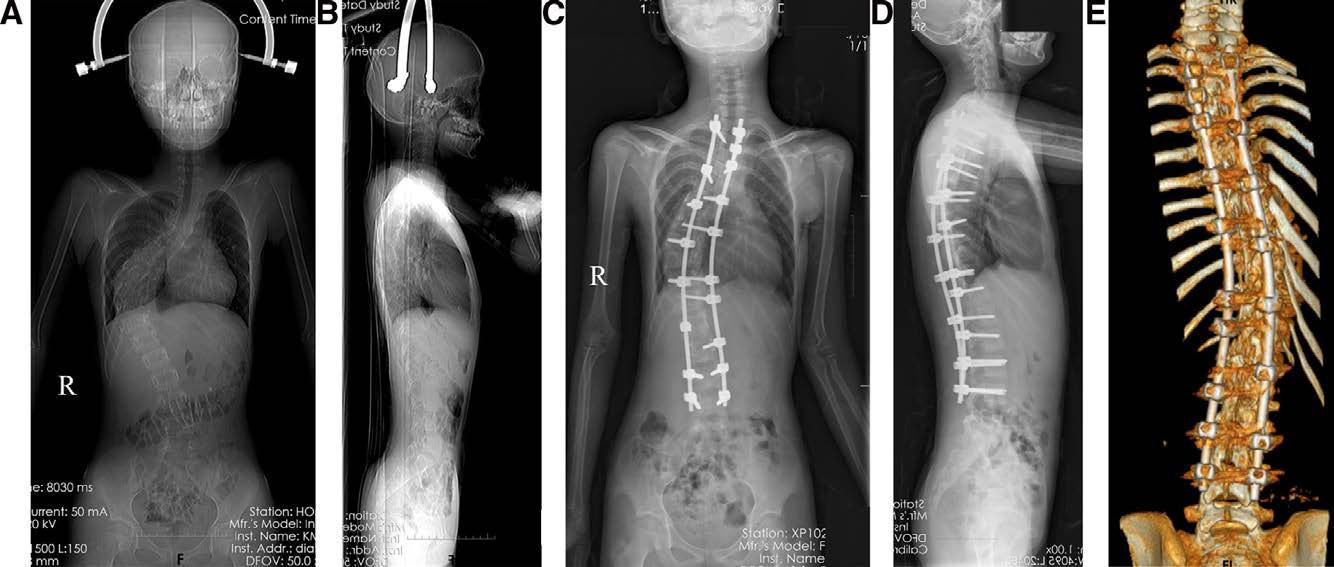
(A) After 15-day skull-femoral traction, an AP X-ray shows an improved Cobb angel (65°), RSH (1.1 cm), and C7-central sacral vertical line (CSVL; 2.6 cm). (B) A lateral X-ray shows an improved Cobb angle (42°) and SVA (0 cm). (C) An AP X-ray taken immediately after surgery, deformity correction, T2-L4 fixation with pedicle screws and rods, and spinal fusion with bone grafts, and thoracoplasty on the convex side. It also shows a Cobb angle of the coronal main curve of 49°, RSH of 1 cm, and C7-CSVL of 2.8 cm. (D) On a lateral X-ray, the Cobb angle is 31°, and the SVA is 0 cm. (E) Postoperative 3-dimensional CT image reconstruction showing posterior spine and implants. AP = anteroposterior, C7-CSVL = C7-central sacral vertical line, CT = computed tomography, RSH = radiographic shoulder height, SVA = sagittal vertical axis.
